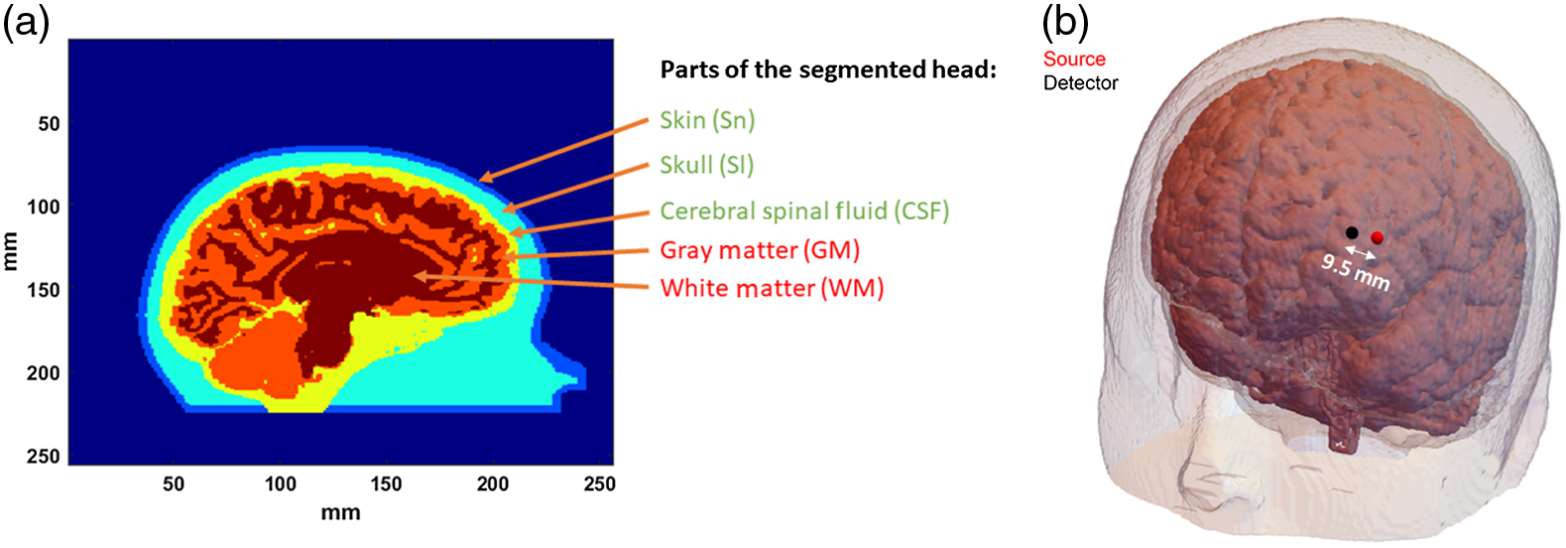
(a) Human head cross section from MRI structural scan, showing different parts of the head. The Sn, Sl, and CSF have been considered extracerebral layers, with GM and WM combined as brain. The dynamics in the Sl and CSF region were considered to be zero. (b) The position of the source and detector locations on the forehead, away from the midline of the brain.
